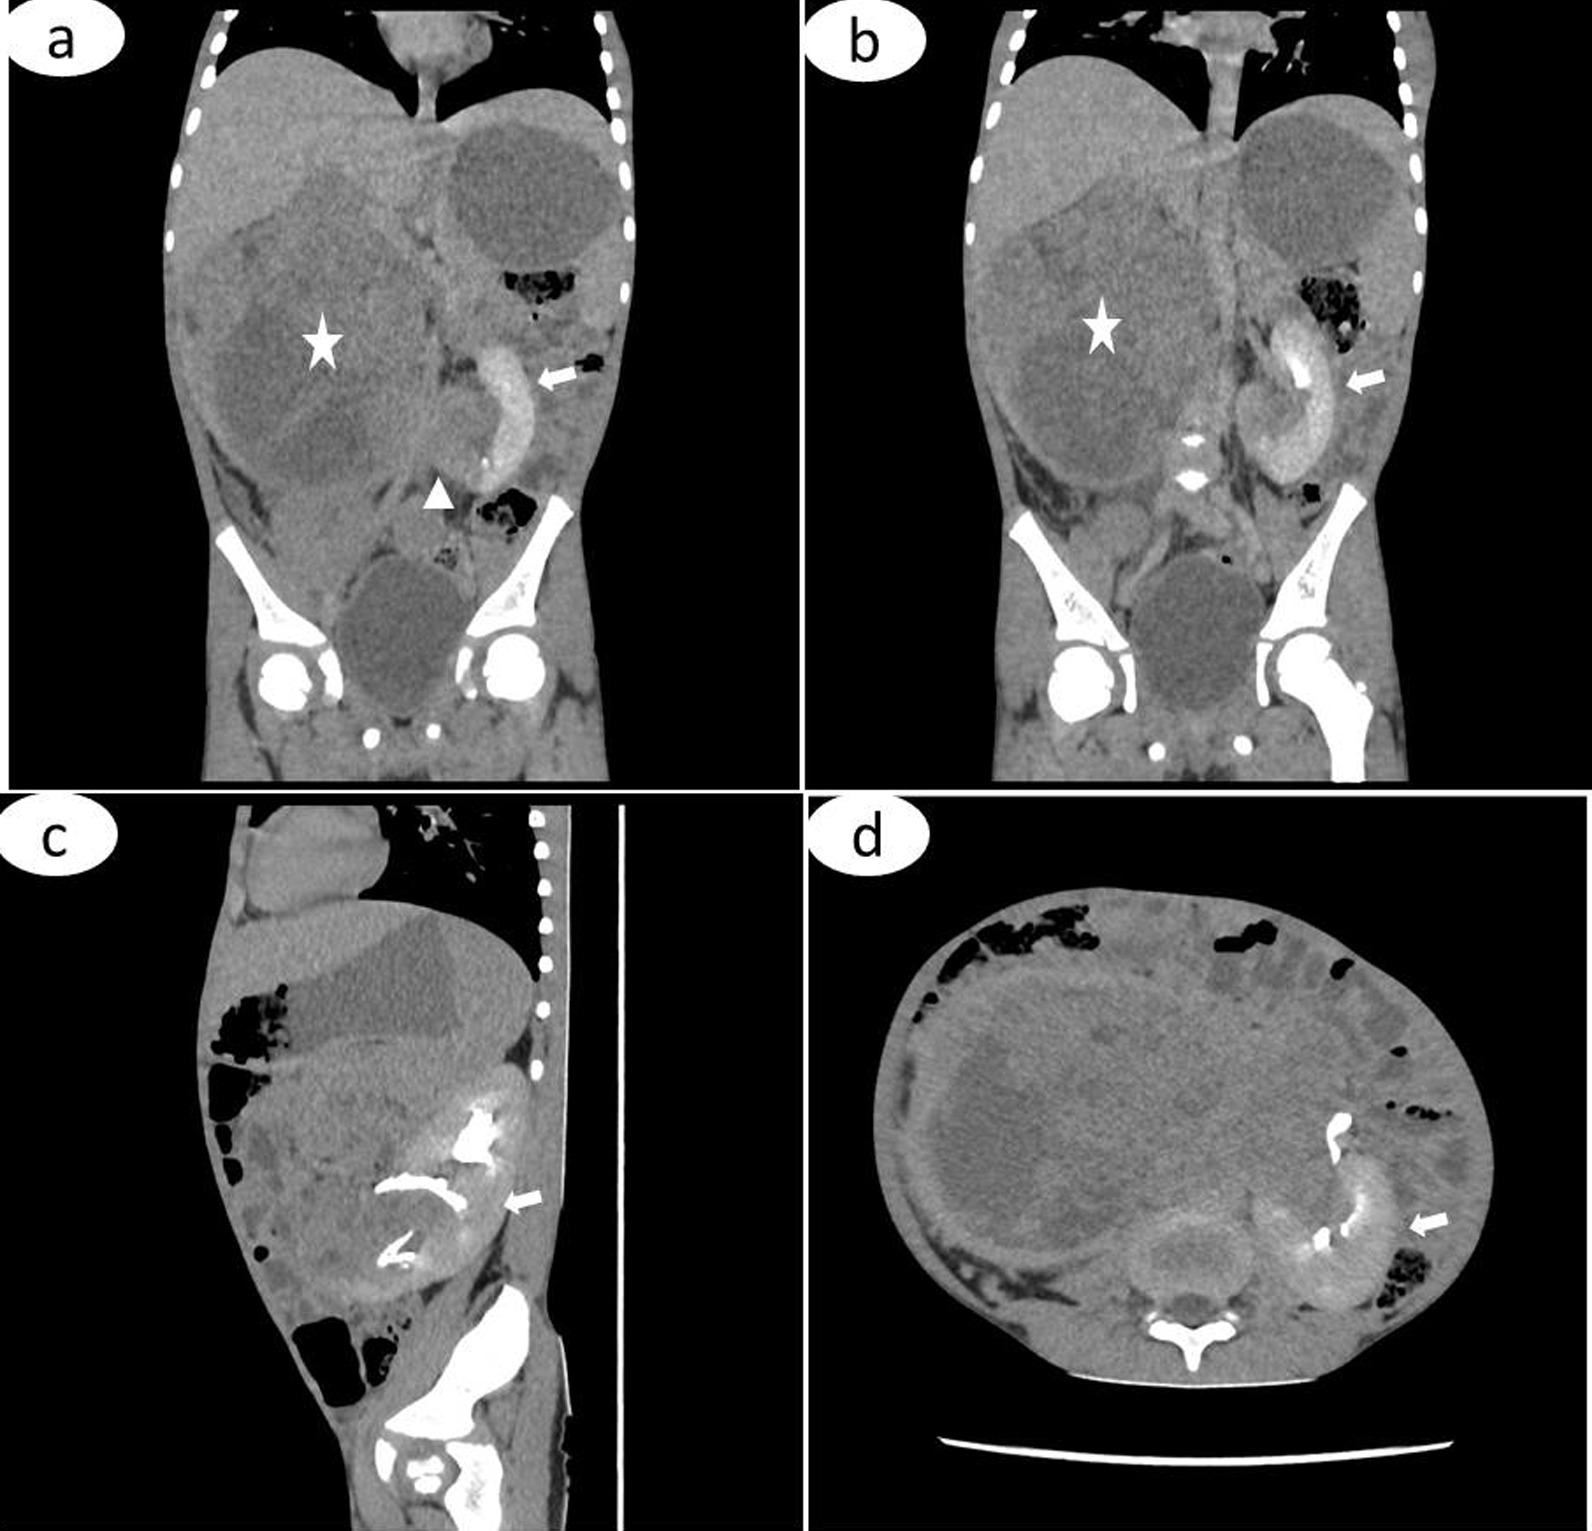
CT images; coronal contrast-enhanced images, anterior to posterior cuts (a, b) showing a heterogeneous non-enhancing low-density mass (asterisks) arising from the right kidney crossing the midline (arrow head) to involve the left kidney (arrows). There is distortion and stretching of the calyces (arrows) of the relatively spared part of the remaining left kidney (c, d)
